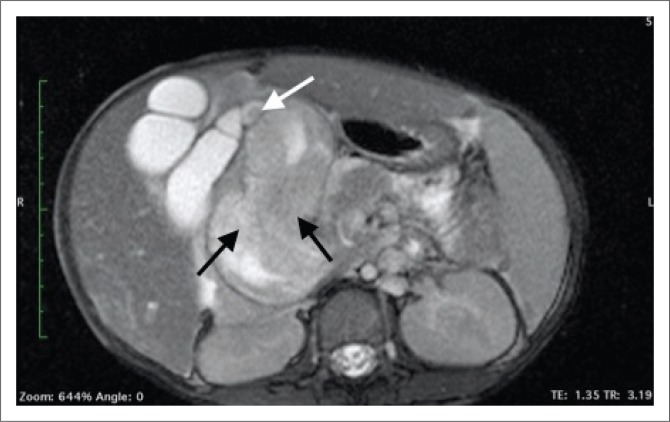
Axial T2-weighted magnetic resonance imaging demonstrating asymmetric mural thickening of the common bile duct (black arrows) with dilation of the gallbladder and a small calculus (white arrow) within the dilated cystic duct.
